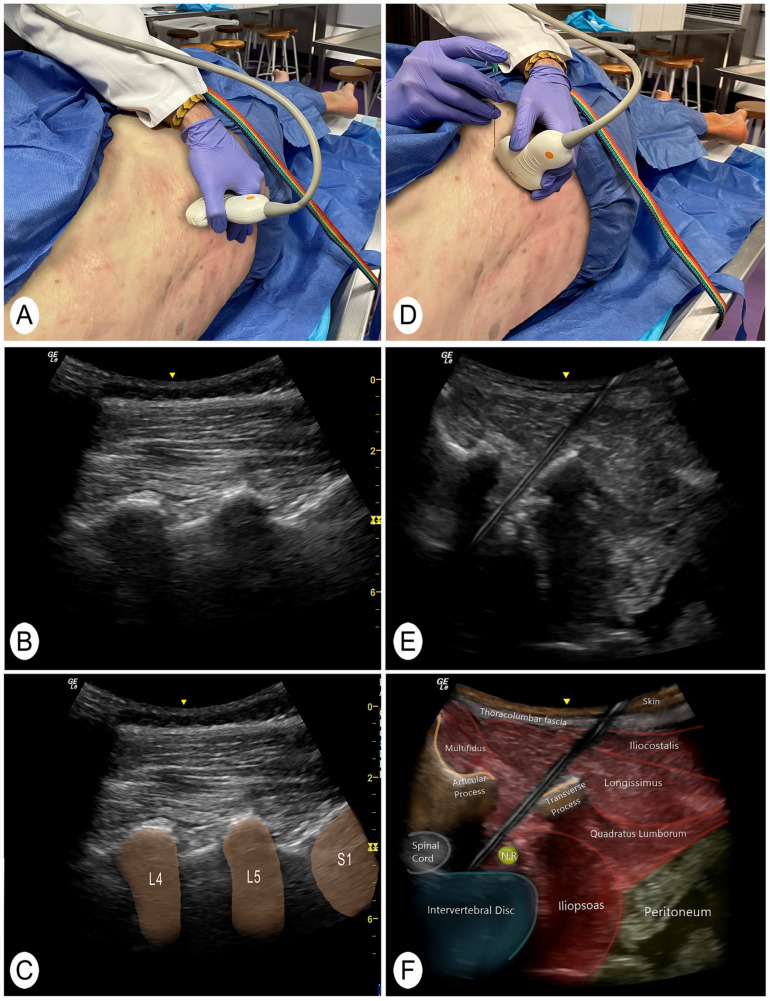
Posterior and lateral ultrasound of the invasive technique. Abbreviations: N.R., nerve root. L4, 4th lumbar vertebra; L5, 5th lumbar vertebra; S1, 1st sacral vertebra. (A) Position to locate the vertebral level with the transducer in a longitudinal slice of the facet joints. (B) Ultrasound image of the longitudinal section of the lumbar articular facets. (C) Ultrasound image of the longitudinal section of the lumbar articular facets with the vertebral segments identified. (D) Position of the transducer to perform the invasive technique in a posterolateral transverse section of the intervertebral disc. (E) Cross-sectional ultrasound image from a posterolateral view where the invasive technique of the intervertebral disc is performed. (F) Ultrasound cross-sectional identification of the structures.
